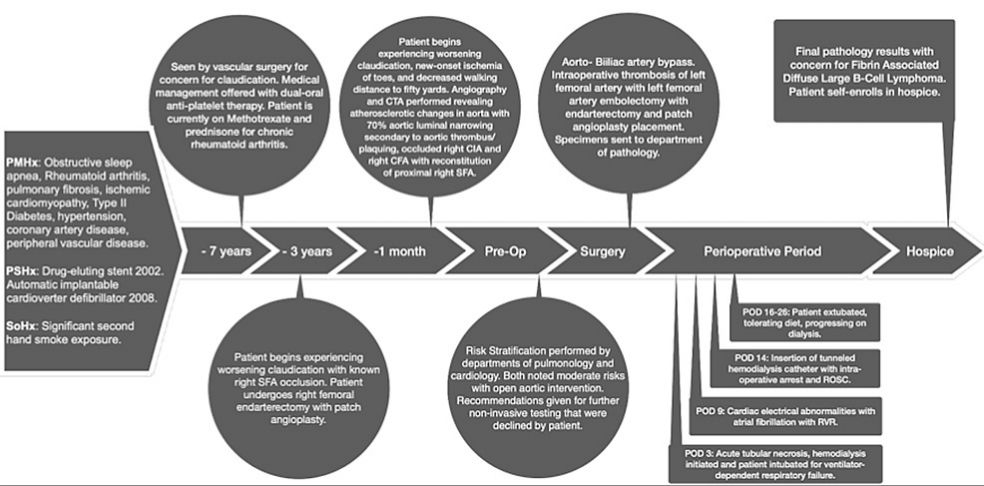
A chronology of notable events throughout patient history. PMHx : past medical history; PSHx: past surgical history; SoHx: social history; CTA: computed tomography angiography; SFA: superficial femoral artery; CIA: common iliac artery; CFA: common femoral artery; Pre-Op: preoperative period; POD: postoperative day; RVR: rapid ventricular response; ROSC: return of spontaneous circulation
